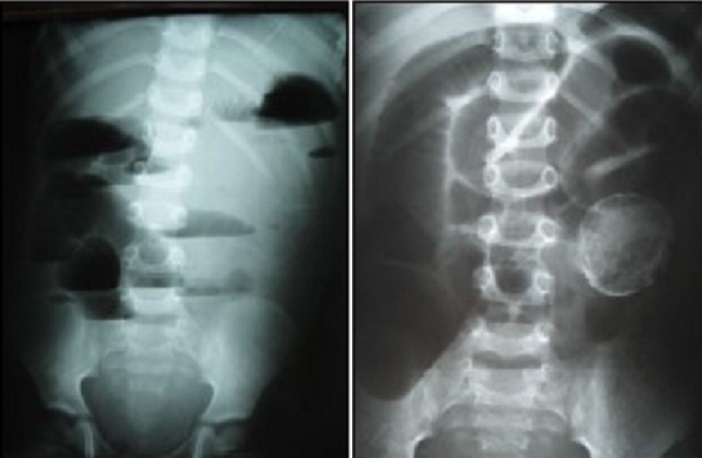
Abdominal plain X-Ray showing multiple air-fluid levels in the small intestine and a radio opaque lesion located at the left lumbar fossa
